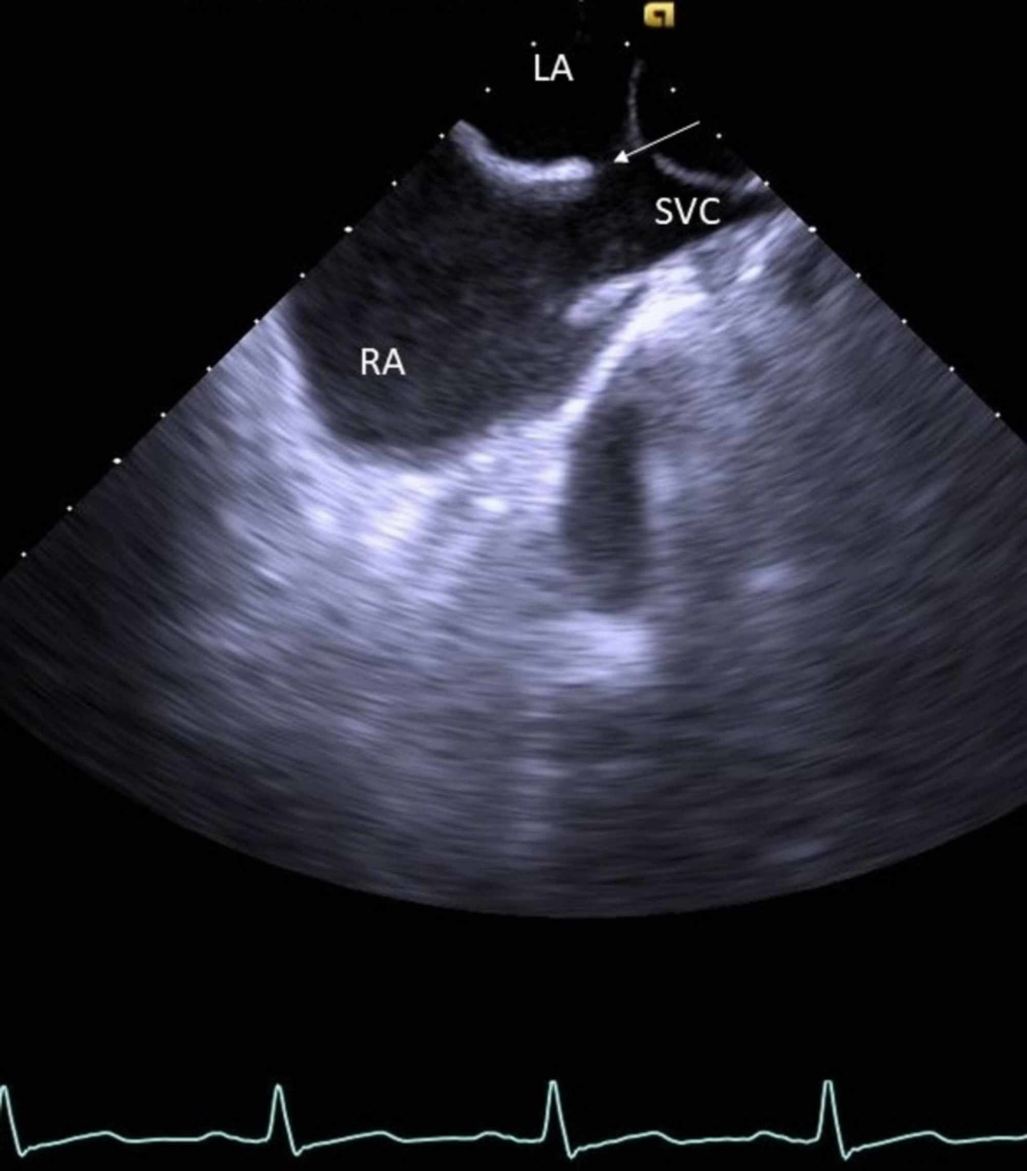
Transesophageal echocardiography The arrow shows superior sinus venosus atrial septal defect. LA, left atrium; SVC, superior vena cava; RA, right atrium
